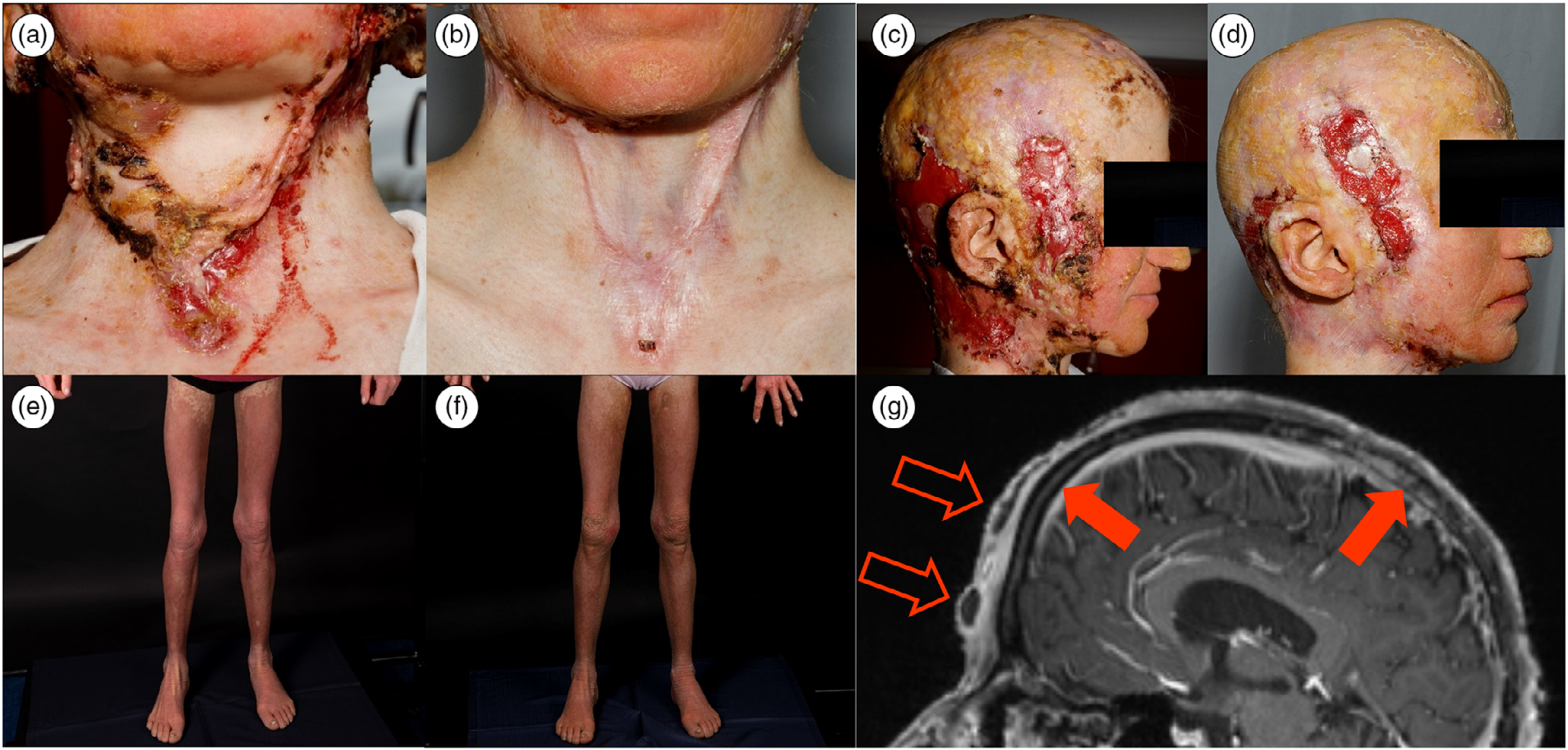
Clinical images (a, c, e) before and (b, d, f) after intervention, and (g) radiological findings. (a) Multiple hyperkeratoses, hypergranulation, and scar strands in the cervical region before surgical intervention and wound care. (b) Wound condition in the cervical region 9 months after surgical intervention. (c) Multiple hyperkeratoses, hypergranulation, and crusts in the periauricular region before surgical intervention and wound care. (d) Wound condition in the periauricular region 9 months after surgical intervention under intensive wound management; note the cicatricial alopecia. (e) Erythrokeratoderma of the legs before initiation of acitretin therapy. (f) Skin condition 6 months after initiation of acitretin therapy, showing a marked reduction in hyperkeratosis and mild decrease of erythema. (g) Sagittal T1‐weighted magnetic resonance imaging after administration of contrast medium showing increased enhancement in the frontal and parietal skull (filled arrows) corresponding to osteomyelitis as well as abscess formations (outlined arrows).
